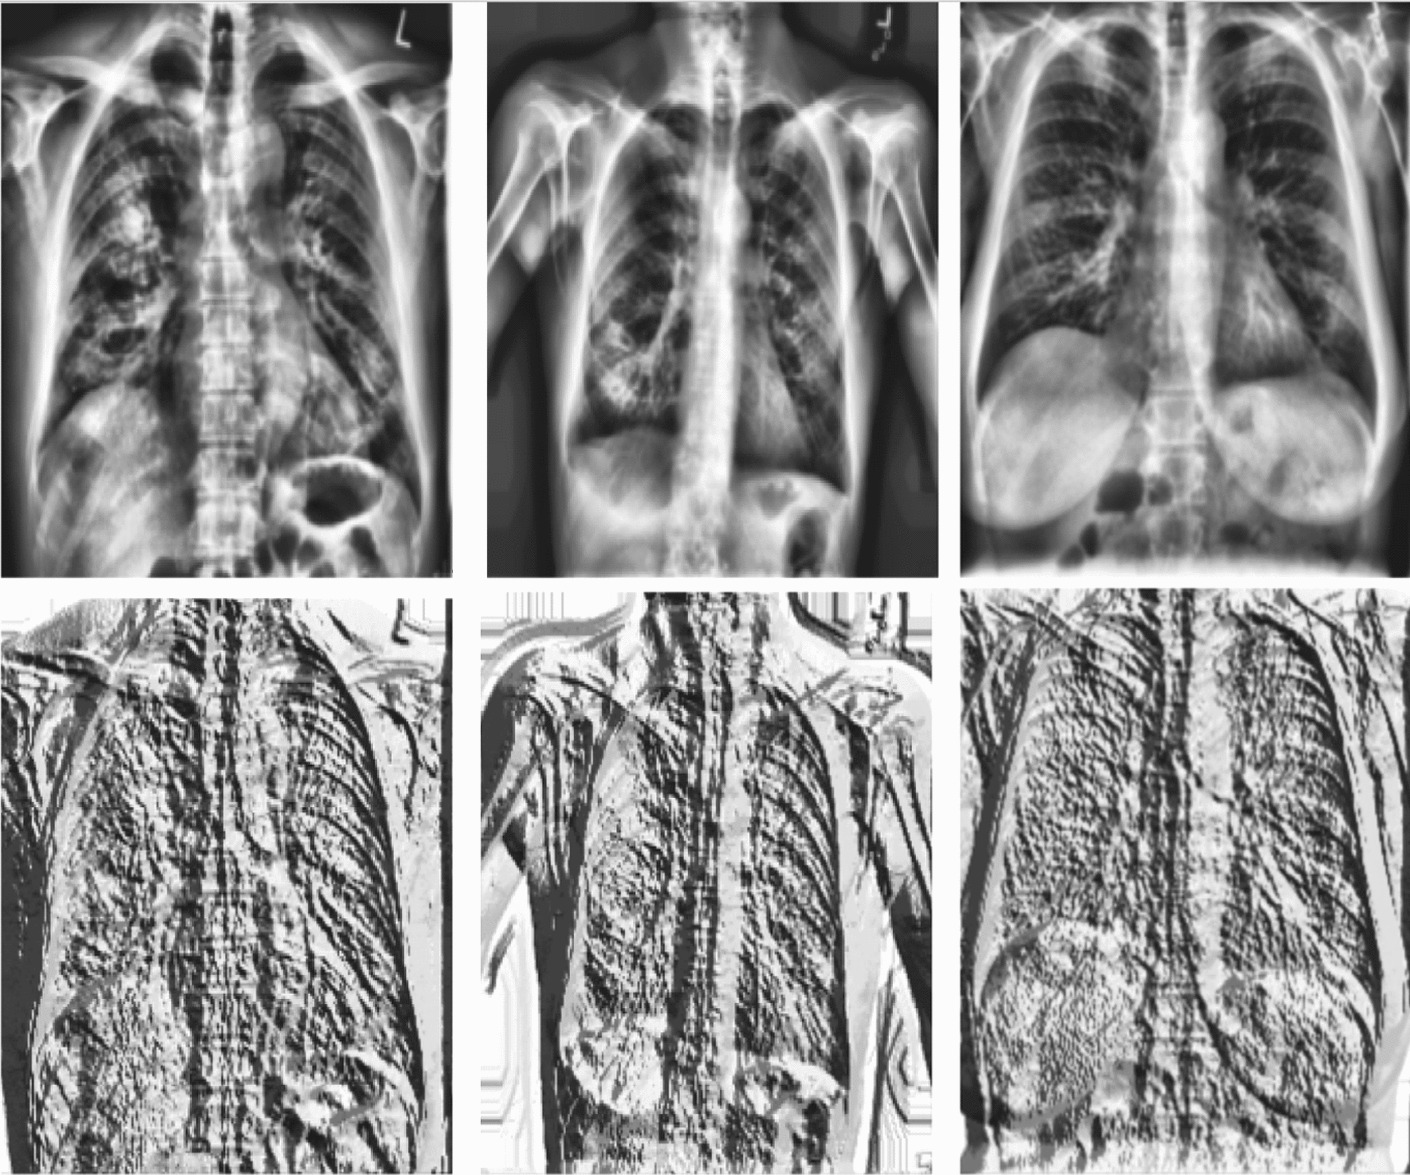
The top first row corresponds to COVID-19, normal, and pneumonia chest X-Ray images. The second row corresponds to the LBP image transformation of COVID-19, normal, and pneumonia
